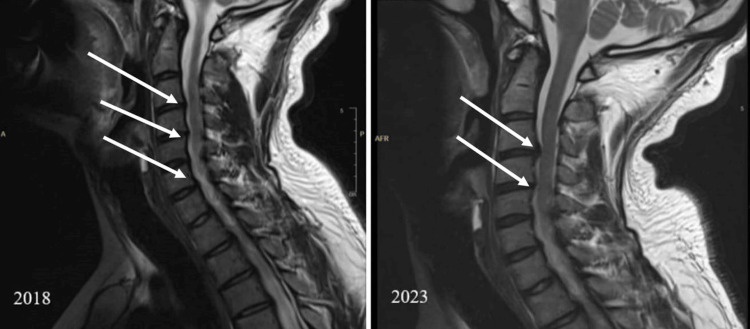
Cervical spine MRI comparison 2018 and 2023 showed a degenerative disc disease at C3-C4, C4-C5, C5-C6, and C6-C7 levels and cervical spondylosis.
